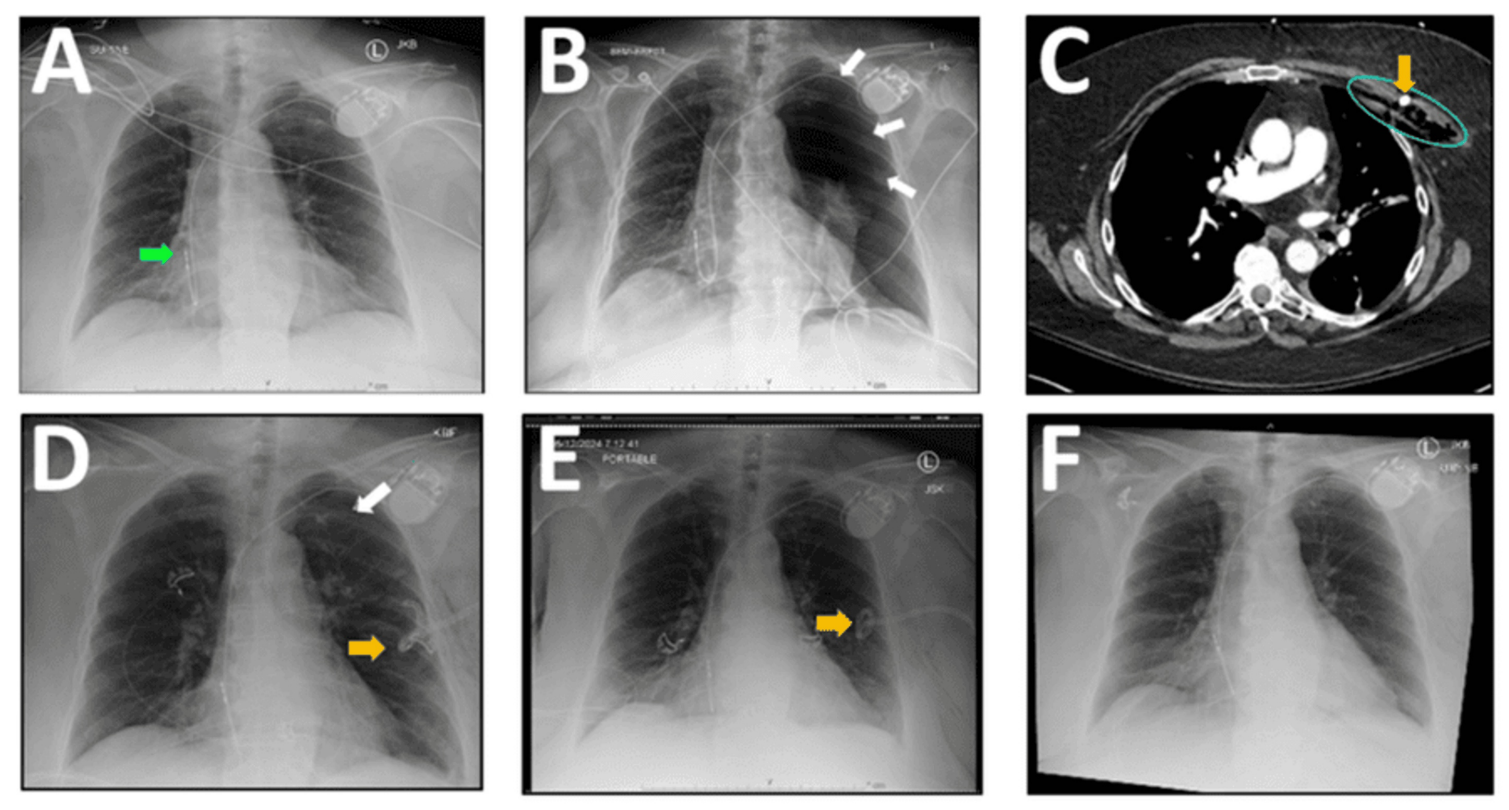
Evolution of pneumothorax Post-op chest X-ray showing the successful placement of a single-chamber atrial pacemaker, with the lead tip (green arrow) indicated (A). The patient presented with pneumothorax (white arrows) five days later (B). CT scan of the chest showed extensive subcutaneous air (blue circle) surrounding the pacemaker generator (C). Pneumothorax was treated with a pigtail catheter (orange arrow), with residual pneumothorax evident on post-op day 3 (D). Resolution of pneumothorax was evident on post-op day 5, indicating that the removal of the pigtail catheter (orange arrow) was appropriate (E). Chest X-ray on post-op day 6 confirmed stable resolution of pneumothorax (F), allowing for patient discharge from the hospital. CT: computed tomography
